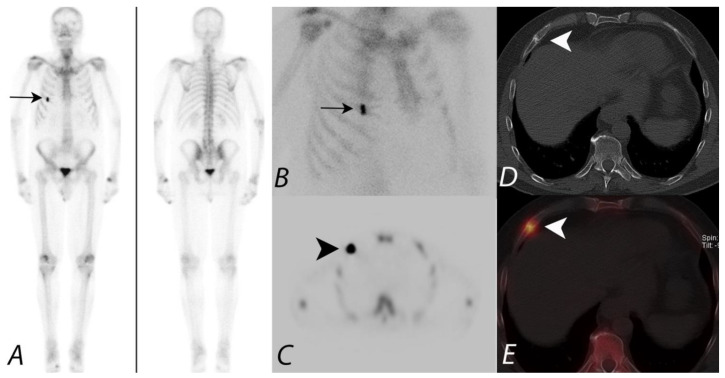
Patient presenting with prostate cancer underwent ^99m^Tc MDP bone scan to evaluate for metastatic disease. Whole body image in anterior projection (A) showing focal increased tracer uptake along the anterior aspect of the right sided 5th rib, confirmed on planar oblique image (B) (black arrows). SPECT image (C) demonstrates focal uptake along the 5th rib anterior aspect (black arrowhead). However, it is unclear if this focus represents a solitary metastatic disease versus another process, such as trauma. Axial CT (D) at the same level provides anatomic information showing a healing fracture (white arrowhead). Fused SPECT/CT image (E) accurately registers the focal activity (white arrowhead) identified on the planar image and SPECT images to the healing fracture on CT image—a great advantage of the hybrid SPECT/CT imaging combining the functional information with anatomic information.
